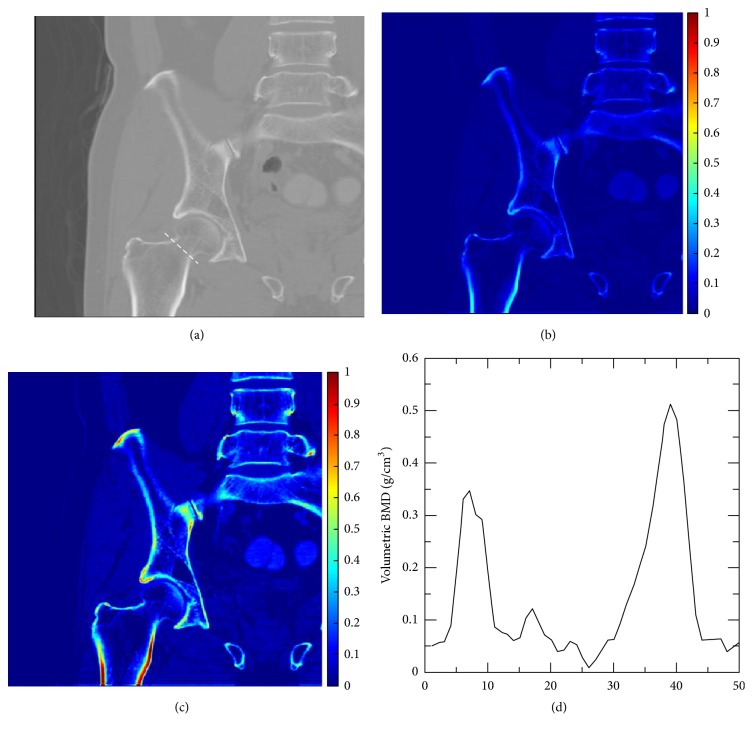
(a) Coronal CT image of a 53-year-old female patient, (b) corresponding BVF map, and (c) vBMD map calculated using the TCM method. (d) The profile of vBMD across the femoral neck showed a nonosseous area in the center of the trabecular bone.
